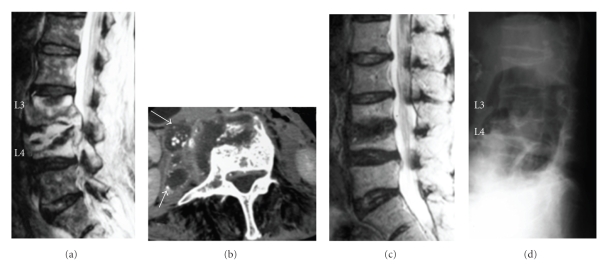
(a) Preoperative MRI in Case 1: T2-weighted imaging. Abscess formation with signal hyperintensity is observed between vertebral bodies and epidurally. (b) Preoperative contrast-enhanced CT. Abscess formation (arrow) is visible around the vertebral bodies, with calcification inside. (c) T2-weighted imaging at 18 months postoperatively. Resolution of abscess is indicated. (d) Plain radiography at 30 months postoperatively. Complete bone union has been achieved.
